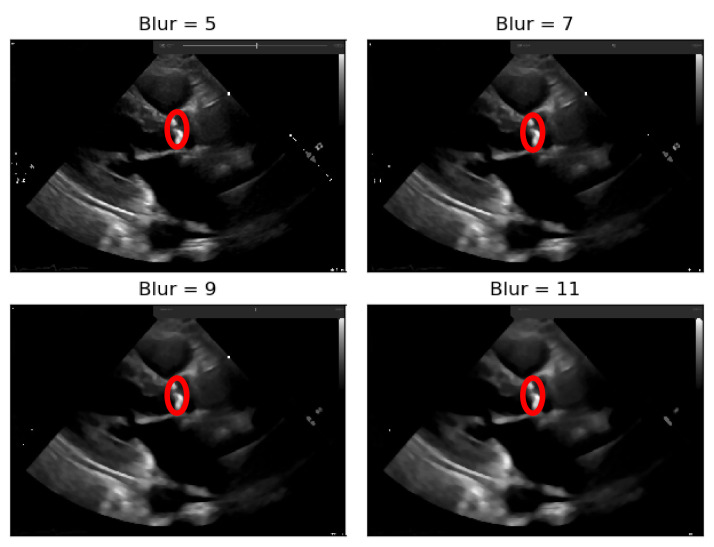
Echocardiography image with four levels of blurring applied—the red circle represents our ROI where the aortic valve is located.
